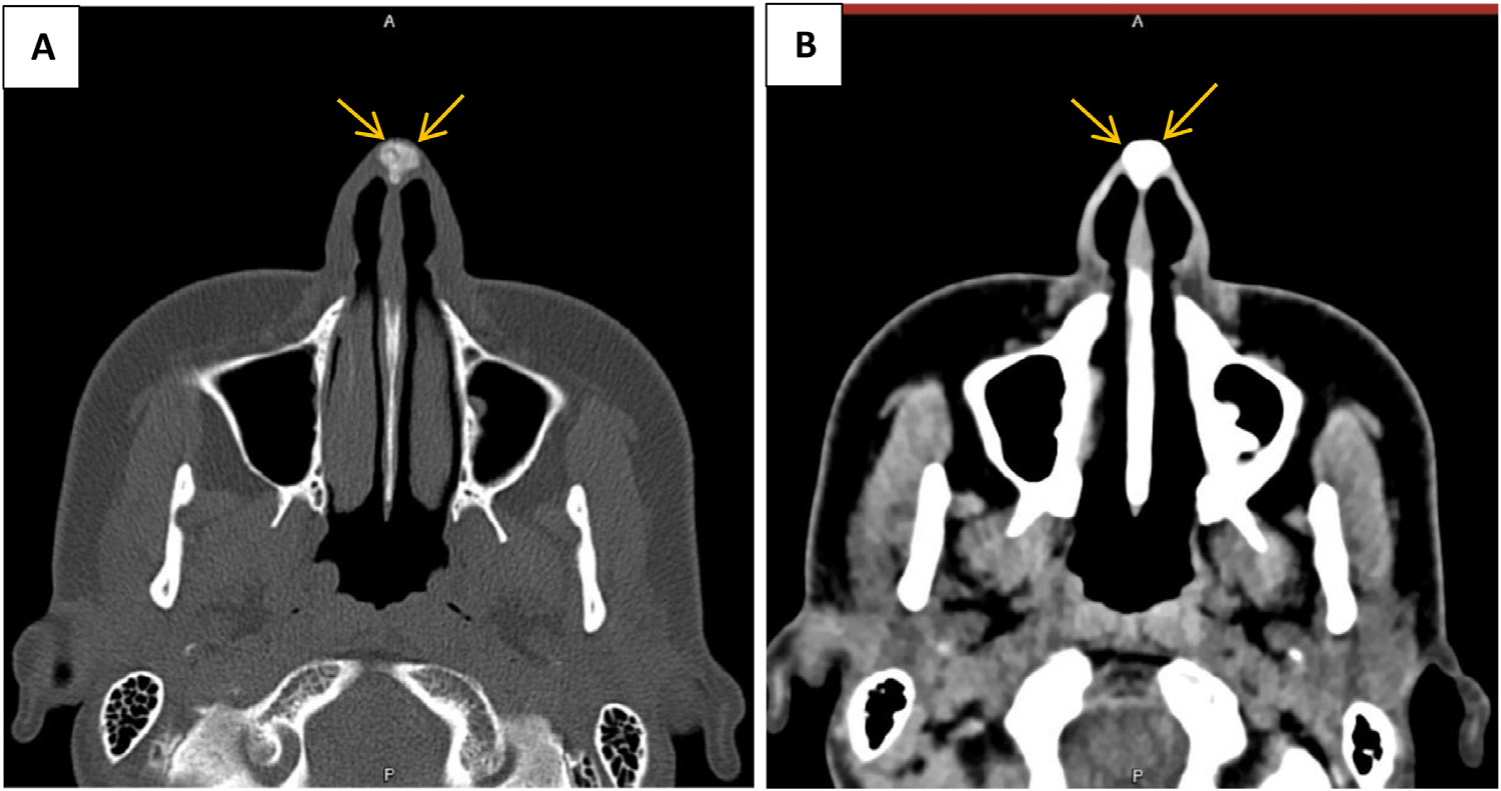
Axial CT scan bone window (A) and soft tissue window (B) shows a hyperdense calcified lesion at nasal tip (Arrows).
